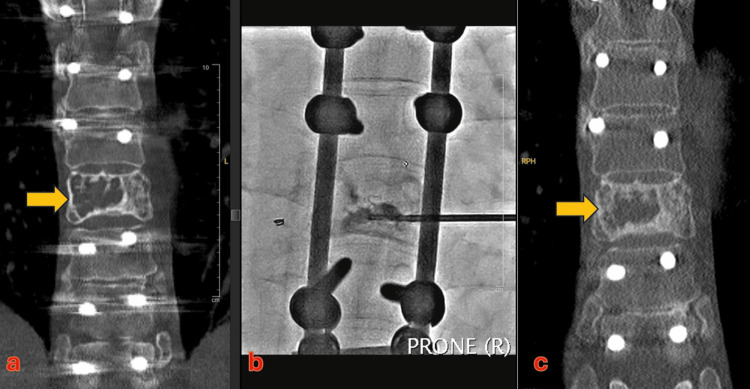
a) Coronal CT reconstructions  demonstrating the recurrent aneurysmal bone cyst (ABC), marked by an arrow. b) An intraprocedure radiograph during percutaneous transpedicular sclerotherapy. c) Note the marked increase in the sclerosis within the previously seen lytic lesion (shown by the arrow) following sclerotherapy.
